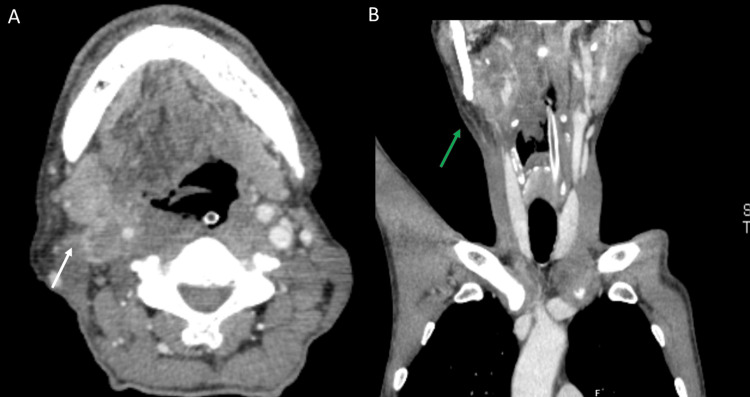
CT scan of the head and neck showing (A) lymph nodes at the right submandibular gland (white arrow), and (B) a heterogeneously enhancing lesion in the region of the right vallecula and base of the tongue (green arrow). CT: Computed Tomography
